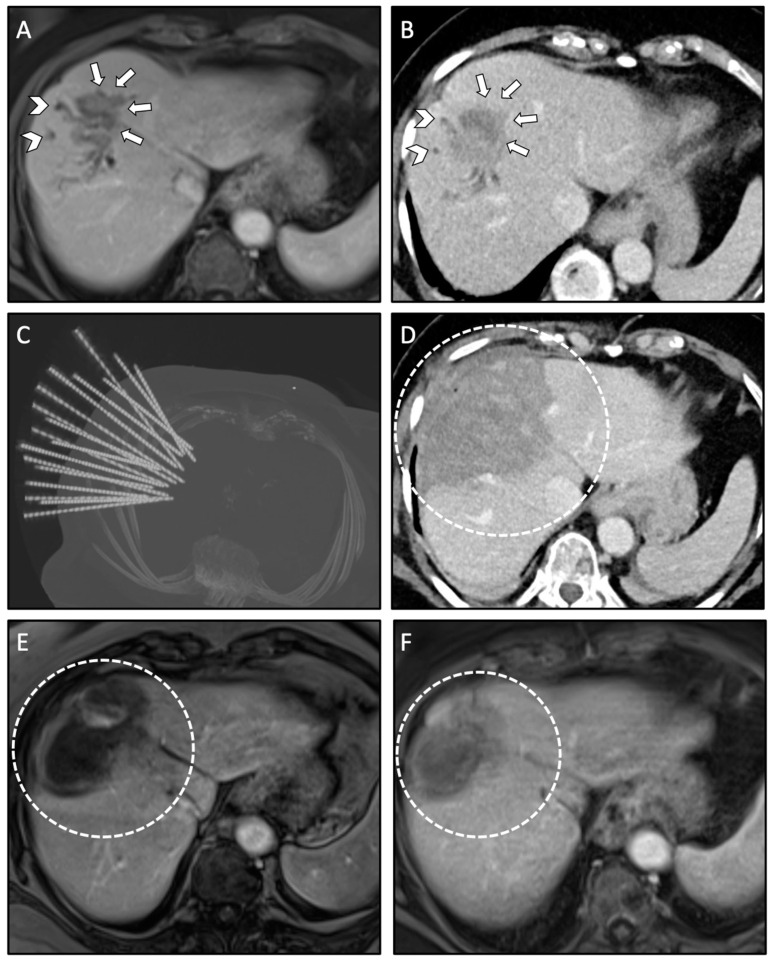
Case of a 76-year-old female with local recurrence of CRLM after conventional US- guided RFA treated by anatomical ablation of liver segment VIII using 18 coaxial needles for SRFA. (A,B) Pre-interventional MRI scan (A) and planning CT scan (B) of the SRFA procedure with 45 mm recurrence of the CRLM (white arrows) and consecutive dilated intrahepatic bile ducts in liver segment VIII indicating cholestasis (white arrowhead). (C) Maximum Intensity Projection (MIP) of the non-enhanced control CT scan showing the position of 18 coaxial needles. (D) Final control CT scan after anatomical ablation with complete necrosis of liver segment VIII. (E,F) Follow-up MRI scan at 3 months (E) and 21 months (F) after SRFA, showing no evidence of LTP with shrinking necrosis zones (white dashed circle).
